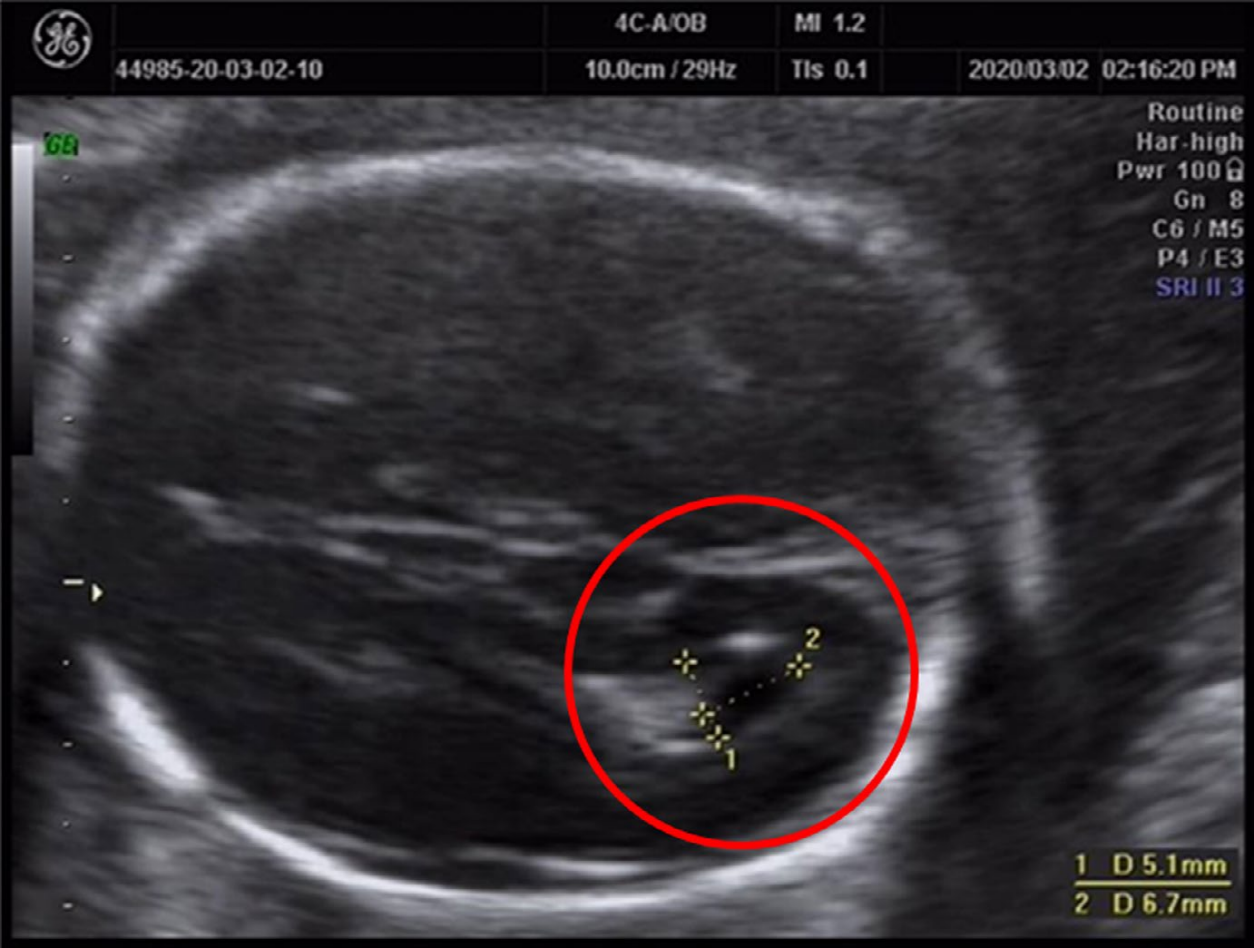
Prenatal ultrasound at 24 wks of gestation shows that the fetus has a right choroid plexus cyst
